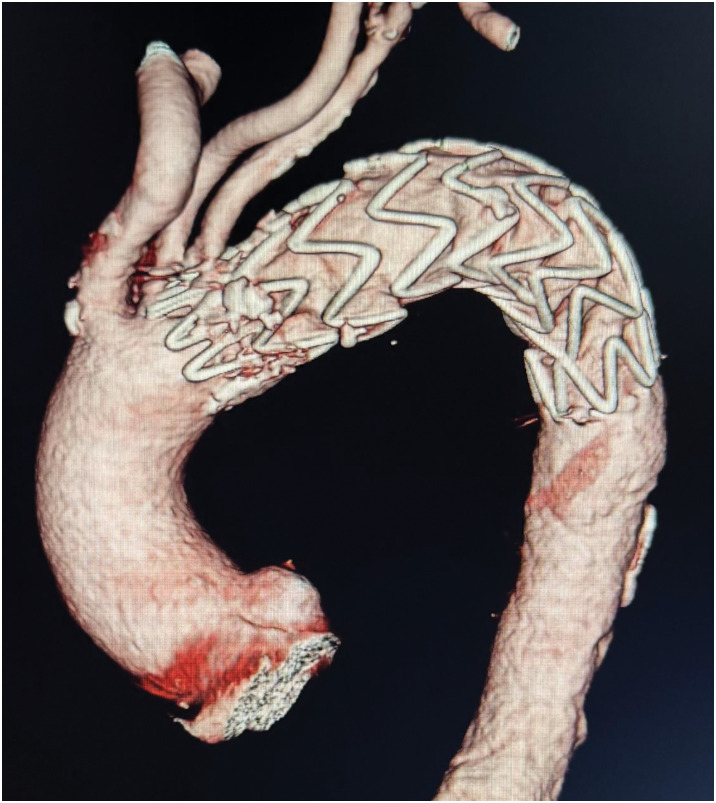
Postoperative contrast-enhanced CT demonstrating complete coverage of the ULP and preserved perfusion of the LSA. CT: computed tomography; LSA: left subclavian artery; ULP: ulcer-like projection
